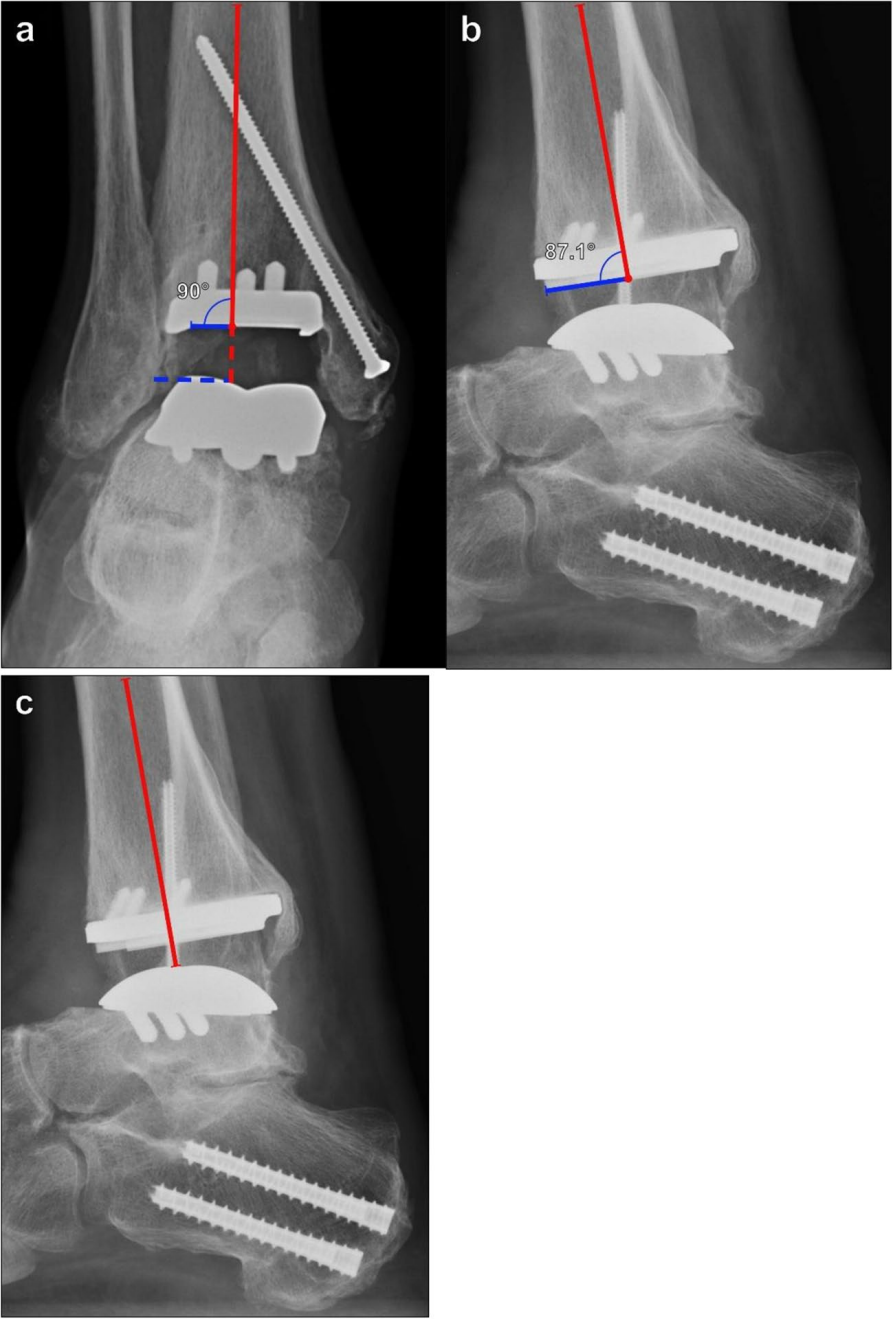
Example of postoperative radiographic appearance of ankle arthroplasty. a On AP radiograph, the articular surface of the tibial component (solid blue line) and axis of the talar component surface (dashed blue line) should be 90° relative to the long axis of the tibia (solid red line). b On lateral radiograph, the articular surface of the tibial plafond component (blue line) should be 89 ± 3° relative to the long axis of the tibia (solid red line). c On lateral view, approximately 40–45% of the talar component should be anterior to the mid-tibial line along the tibial axis (red line). These parameters help to identify device malalignment or early component migration
